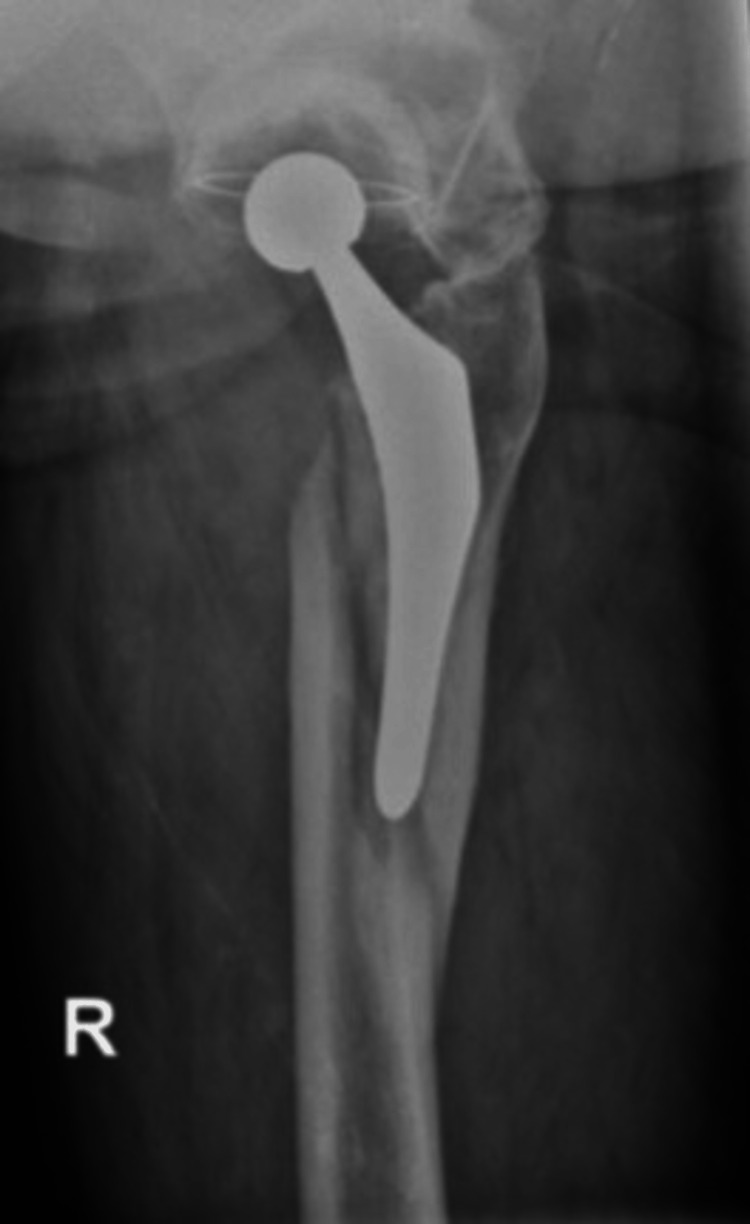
Case B, right X-hip-axial pre-OR X-ray showing a periprosthetic femoral fracture of the right femur, Vancouver B1 type of fracture
